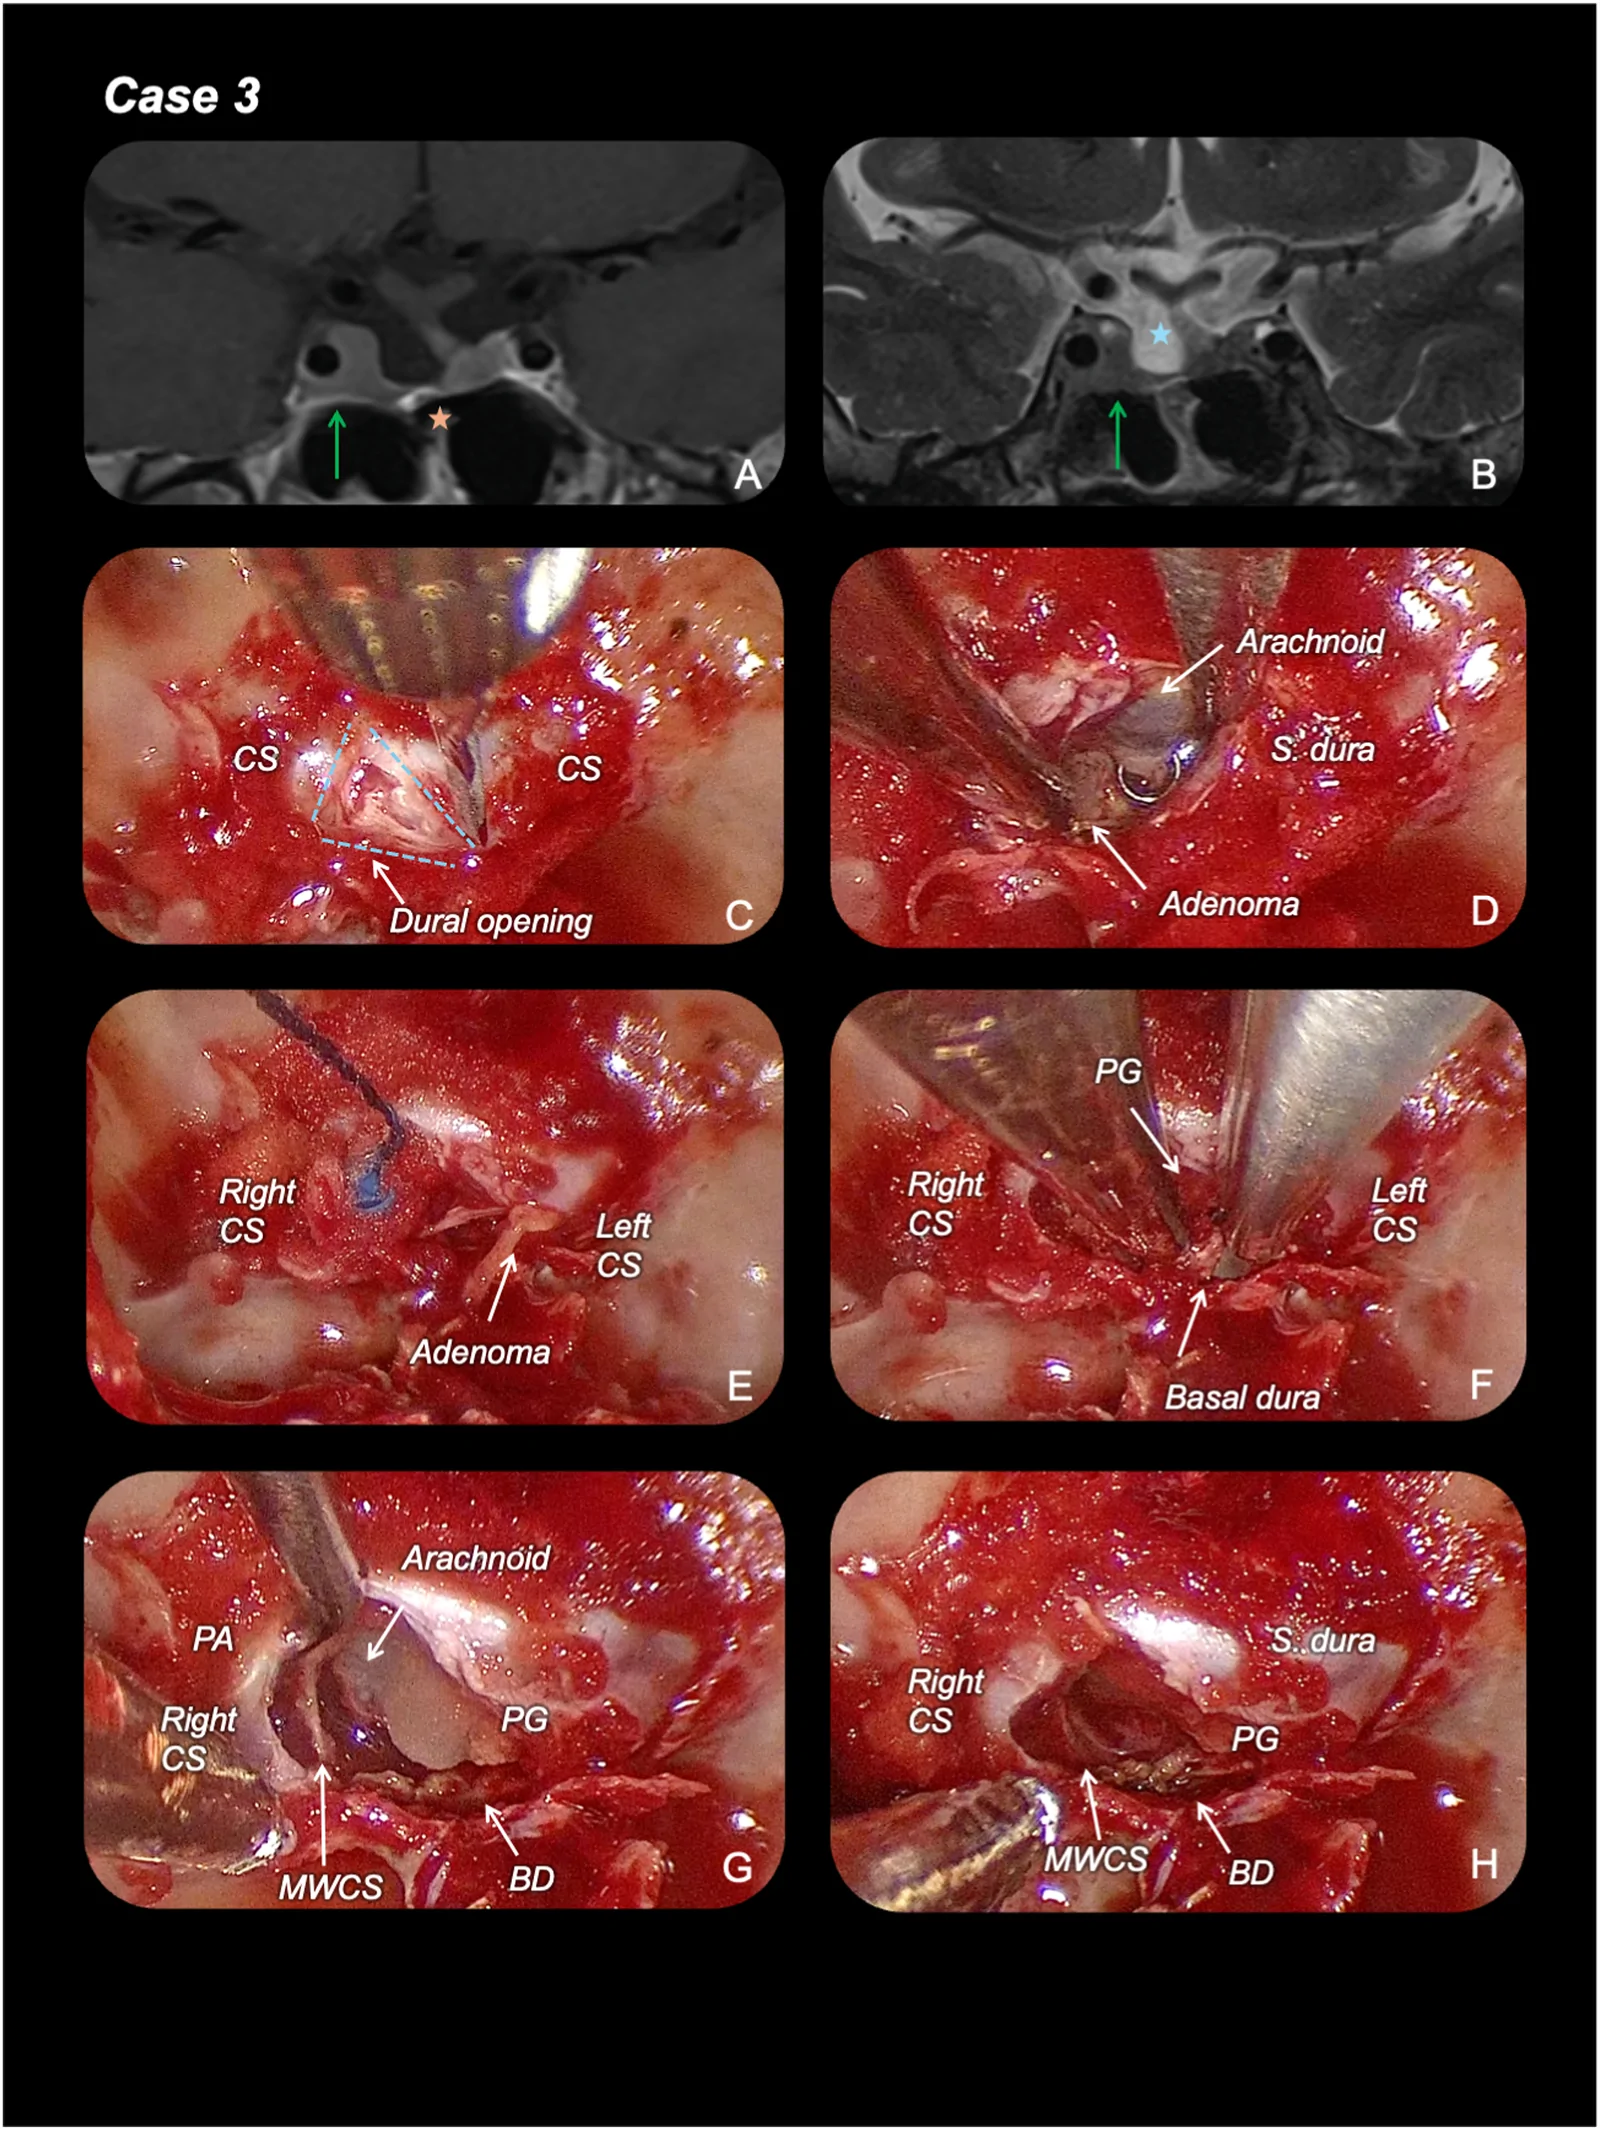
MRI-positive with a macroadenoma, basal dura and cavernous sinus invasion (Case 3) (A–B) Preoperative coronal T1-and T2-weighted MRI showing an 11-mm right-sided adenoma (green arrows), Knosp grade 3B. The orange star indicates basal dura (BD) invasion, while the light-blue star indicates prolapse of the sellar diaphragm. (C–D) Triangular dural opening performed to avoid subarachnoid space opening. Initial partial tumor removal was achieved on the right side. (E–F) During right cavernous sinus (CS) hemostasis, resection of the adenoma and involved basal dura on the left paramedian side. (G–H) Interdural dissection with opening of the medial wall of the cavernous sinus (MWCS), followed by piecemeal intracavernous tumor resection using a curette. Subtotal resection was achieved due to adherence to the right internal carotid artery (ICA). *Abbreviations*. PA: pituitary adenoma; PG: pituitary gland; S.dura: sellar dura.
